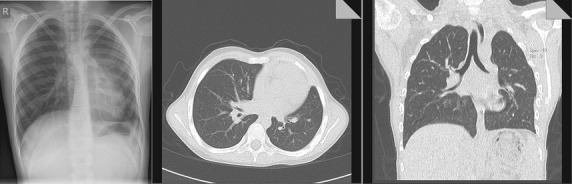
Chest X-ray (anterior–posterior scan) and chest CAT scan (axial and anterior–posterior scan) of CaseID 3279 with *STRA6* mutation show an elevated diaphragm, a right–left lung asymmetry with reduced lung volume in the left lung, and left-shifted heart.
